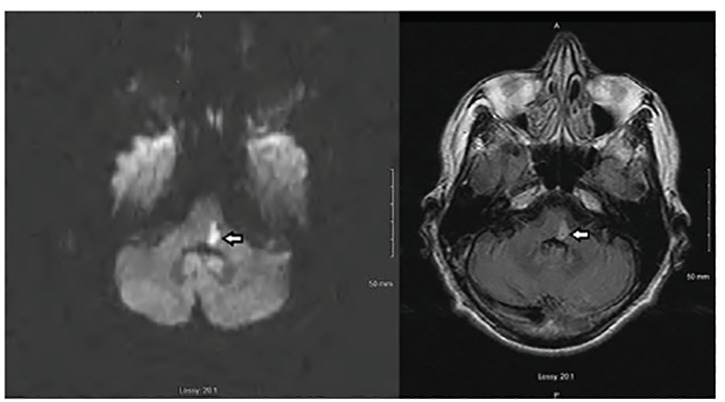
Magnetic resonance images showing left medial pontomedullary junction infarction. Axial diffusion-weighted image (left) and fluid-attenuation inversion recovery image (right) demonstrate the acute ischemic lesion. ⇦ Site of infarction.
